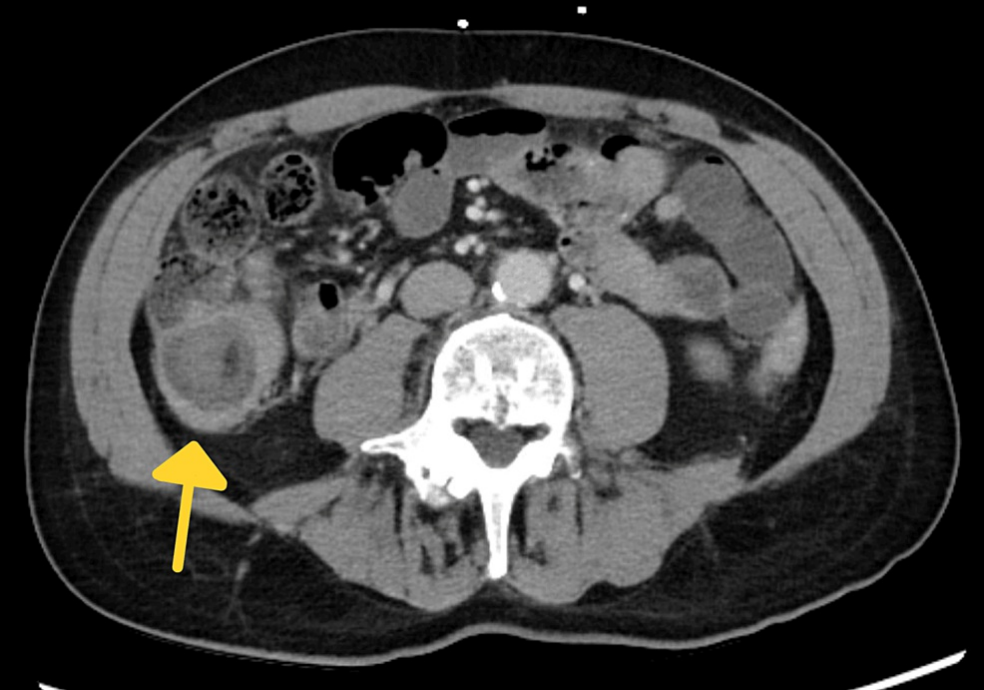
CT showing target sign of small bowel obstruction
